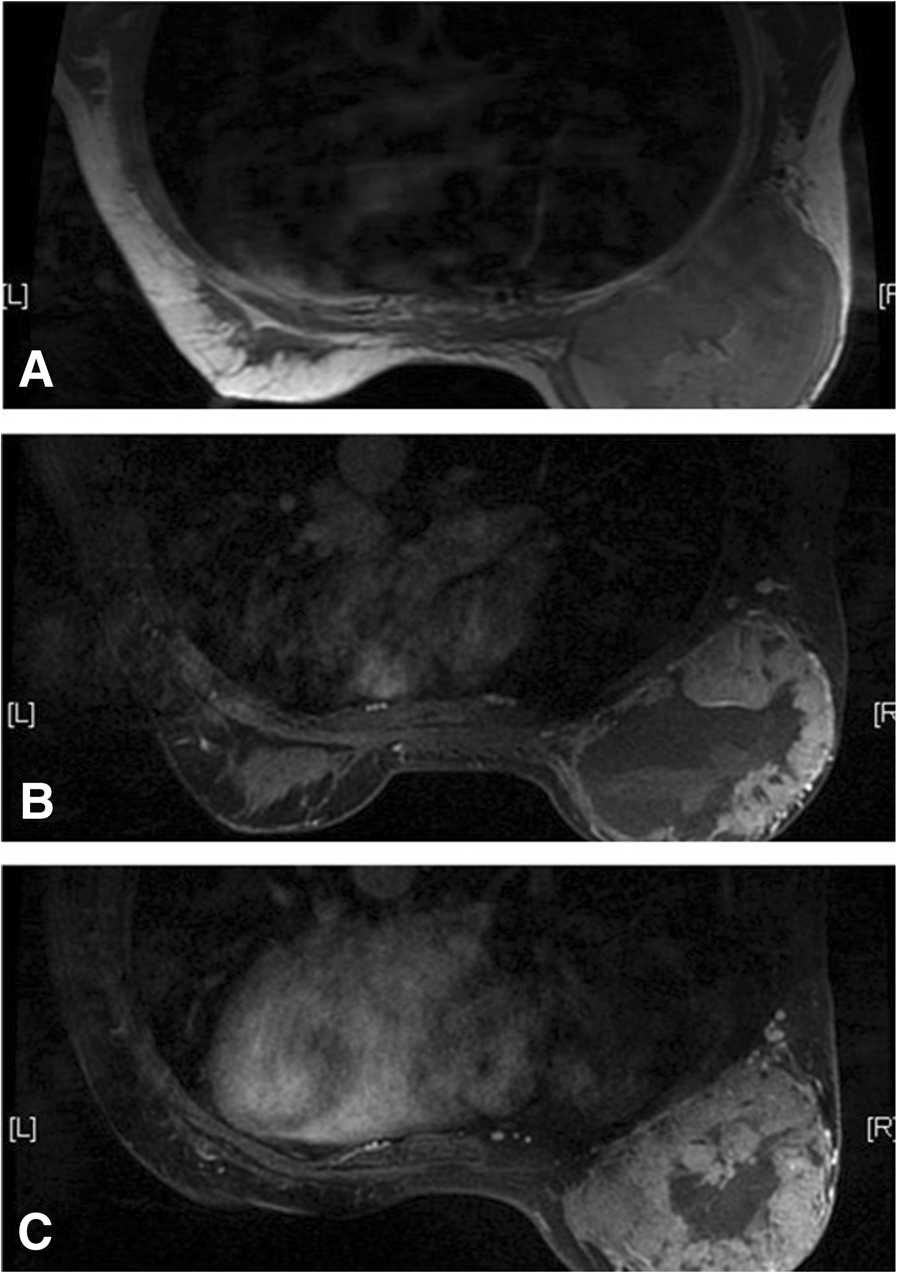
A female whose right breast has been swollen for 1 months. The mass has been enlarging in a high rate since she was pregnant recently. a: On T1-weighted images, the hemorrhagic lesion shows equal and high signal intensity. b: The contrast enhanced images demonstrate significant and heterogeneous enhancement in the early stages. c: Concentric enhancement of the contrast agent is observed in the delayed phase
